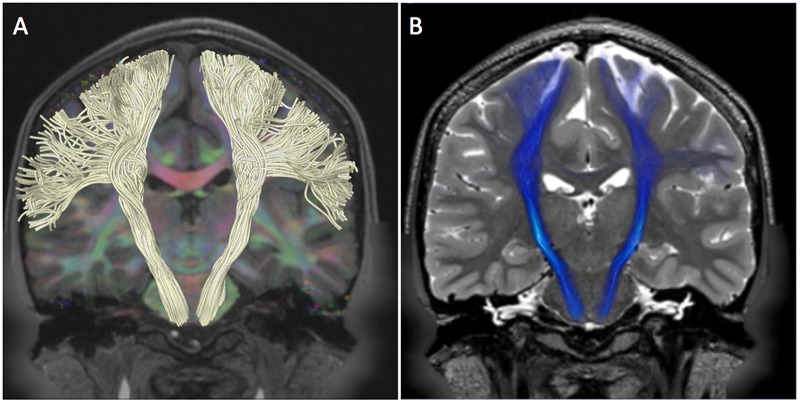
Pyramidal pathway in HCP subject 100307. The figure shows the tractography reconstruction of pyramidal white matter fibers arising from the pre-central gyrus. (A) Pyramidal pathway (white) reconstructed using the MC-det approach. The tracts are displayed on a DEC map overlaid on a coronal T1-weighted image. (B) Pyramidal pathway (blue) reconstructed using the CSD-prob approach. The tracts are displayed on a coronal T2-weighted image.
